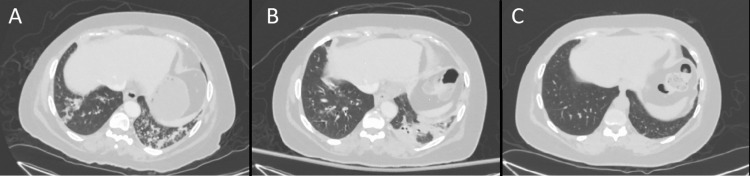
CT chest CT: computerized tomography scan; A: CT chest upon presentation showing bilateral pneumonia with the largest consolidation in the left lower lobe, B: follow-up CT chest a few days after presentation showing persistence of bilateral pneumonia, C: follow-up CT chest two months after presentation showing resolution of bilateral pneumonia.
